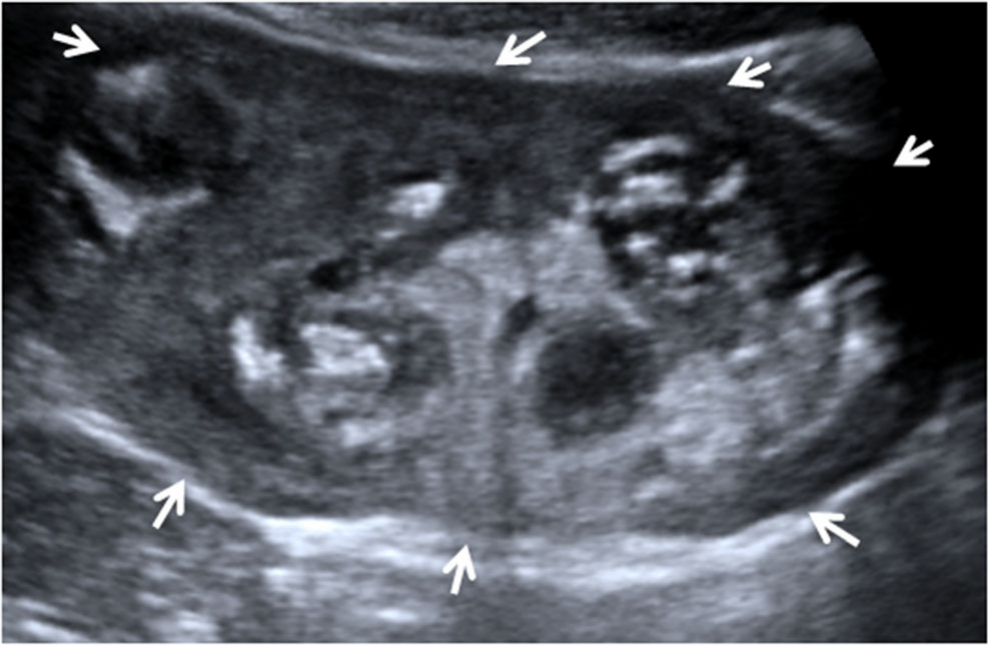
Diffuse XPN. Longitudinal sonogram image shows a large kidney maintaining reniform shape with heterogeneous content (*arrows*)
